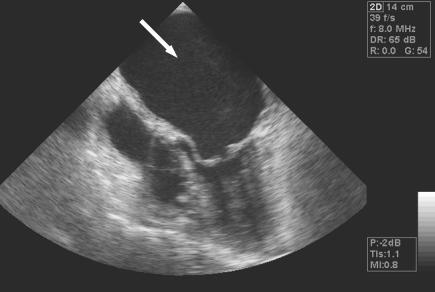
Trans-Esophageal Echocardiogram (TEE) TEE view of significant mitral stenosis (thickening of the mitral valve leaflets with doming of the anterior leaflet) with severe spontaneous echo contrast (white arrow).
